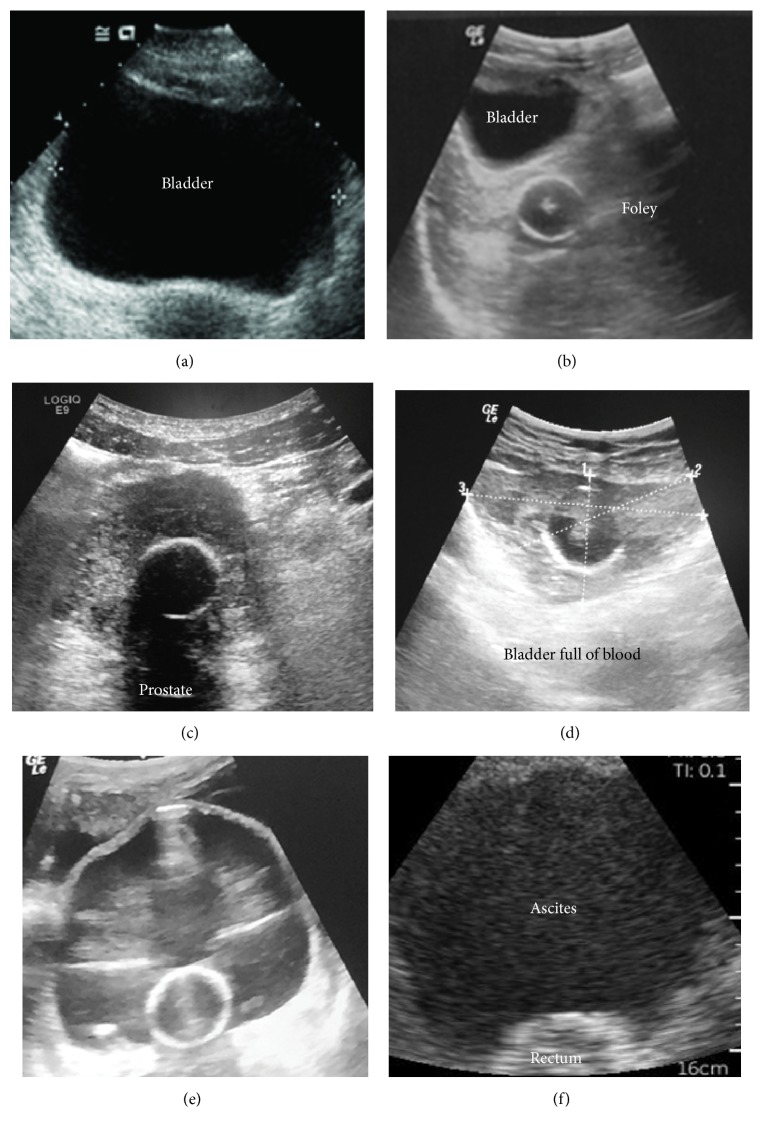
Postrenal assessment: bladder. (a) To calculate bladder volume, the maximum anterior-posterior bladder diameter is measured on an axis perpendicular to that of the longitudinal measurements. Volume (mL) = length (cm) × width (cm) × height (cm) × (0.52 to 0.57) for an ellipsoid (see Figure 4, probe position A). (b) shows a Foley bulb deployed in the pelvis of a patient with anuric renal failure. (c) shows a Foley bulb inflated in the prostate. (d) shows a Foley catheter positioned in a bladder filled with coagulated blood of an anuric patient. (e) Bladder is distended around the Foley bulb due to catheter obstruction. (f) Patient with ascites. Suprapubic view is sensitive for detecting pelvic fluid. It may be difficult to differentiate bladder fluid from ascites with ultrasound.
